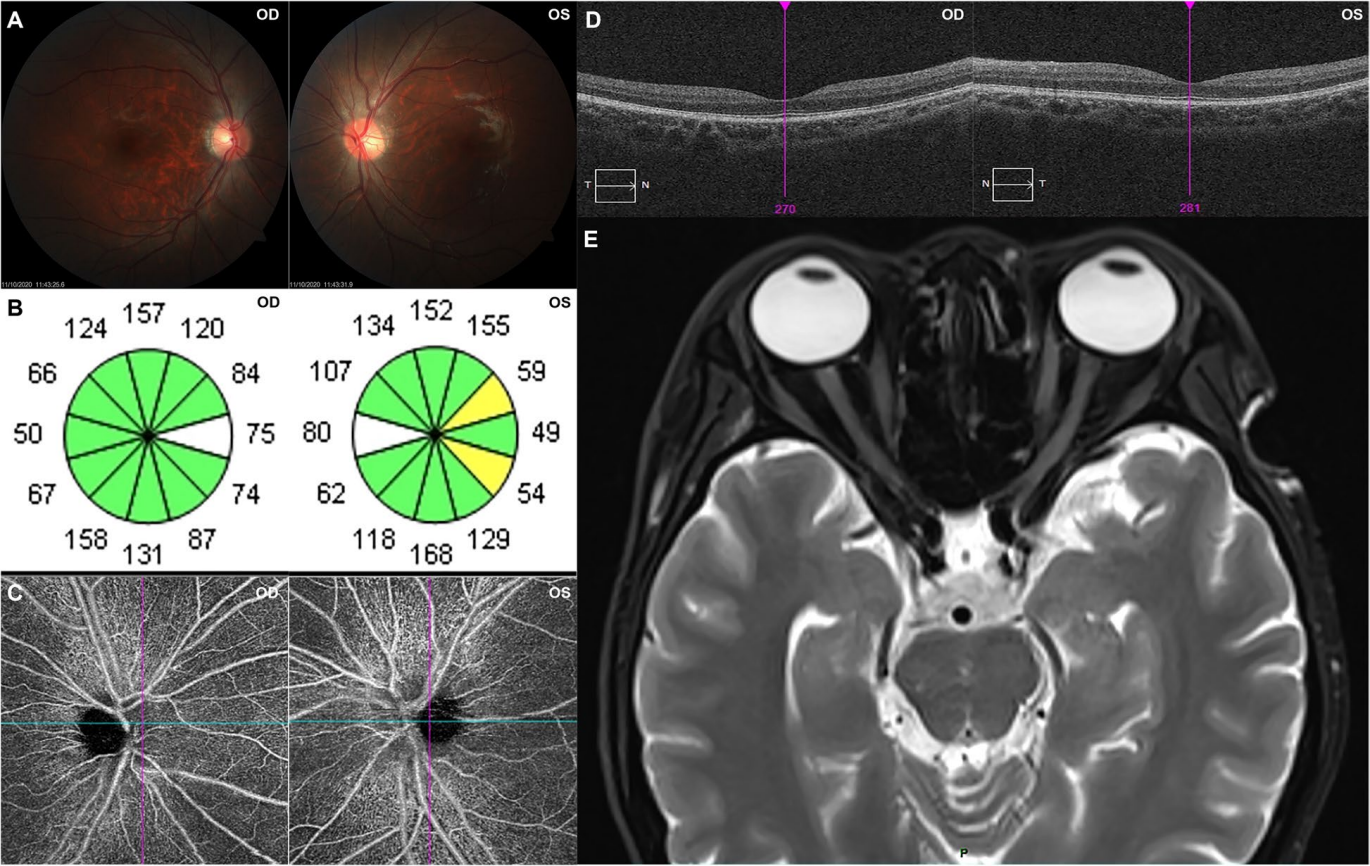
Ocular examinations and MRI. Fundus photography A showed optic disc with normal morphology. OCT B showed normal RNFL thickness. OCTA C showed normal capillary perfusion density. Macular OCT D showed normal morphology. MRI E showed no noticeable abnormality in optic nerve and brain. Abbreviations: OCT, optical coherence tomography; RNFL, retinal nerve fiber layer; OCTA, optical coherence tomography angiography; MRI, magnetic resonance imaging; OD, oculus dextrus; OS, oculus sinister
